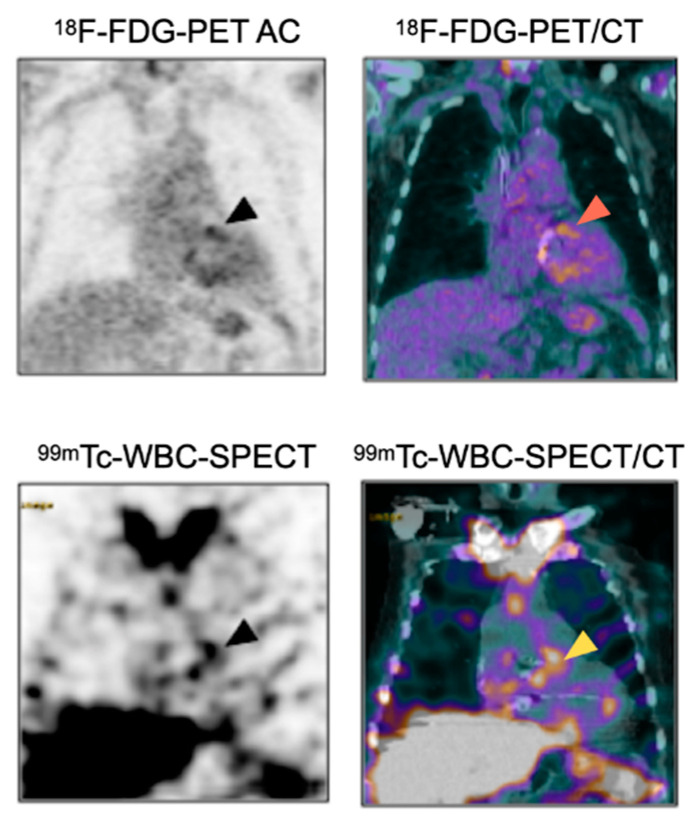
Complementary diagnostic value of ^18^F-FDG-PET/CT and ^99m^Tc-WBC-SPECT/CT for the diagnosis of PVE. Patient with suspicion of aortic PVE one month after surgery. ^18^F-FDG-PET/CT showed intense uptake around the aortic prosthetic valve (arrowheads), which could in this context be related to PVE or to post-surgery inflammatory reaction. ^99m^Tc-WBC accumulation in the same area as the ^18^F-FDG-PET/CT helped to confirm the diagnosis of prosthetic valve infection.
